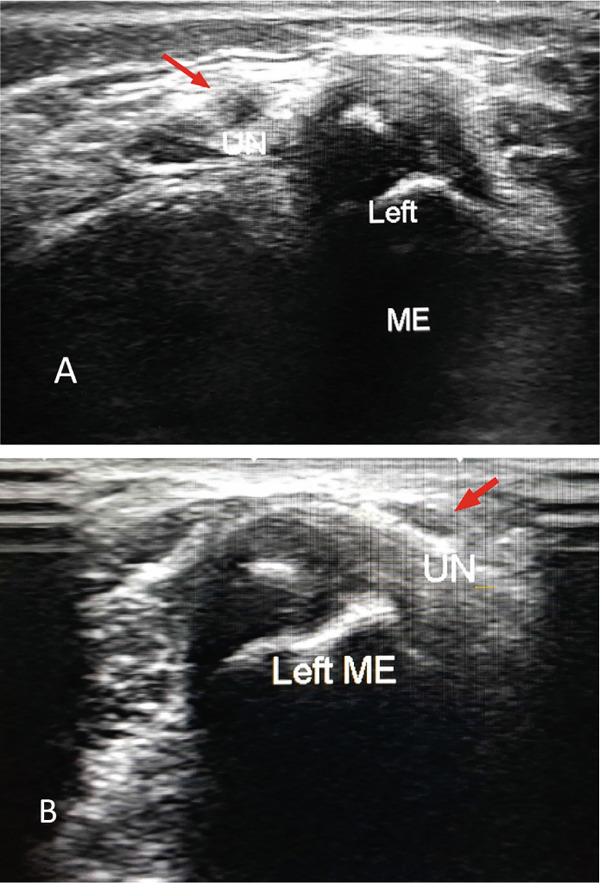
Axial ultrasound image of the contralateral normal elbow; (A) the ulnar nerve (red arrow) lying just posterior to the tip of the medial epicondyle on elbow extension, (B) the ulnar nerve (red arrow) dislocated anterior to the medial epicondyle on elbow flexion.
